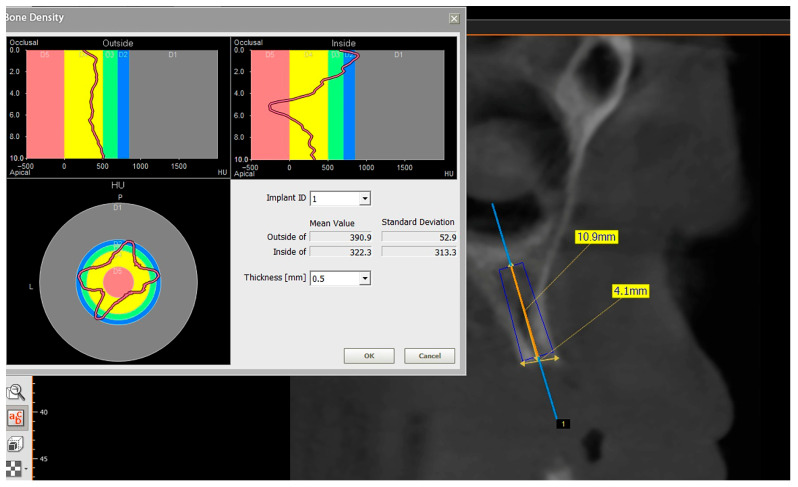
Bone density around a virtually placed dental implant—CBCT (Green X™ 12^®^, Vatech, Prague, Czechia).
